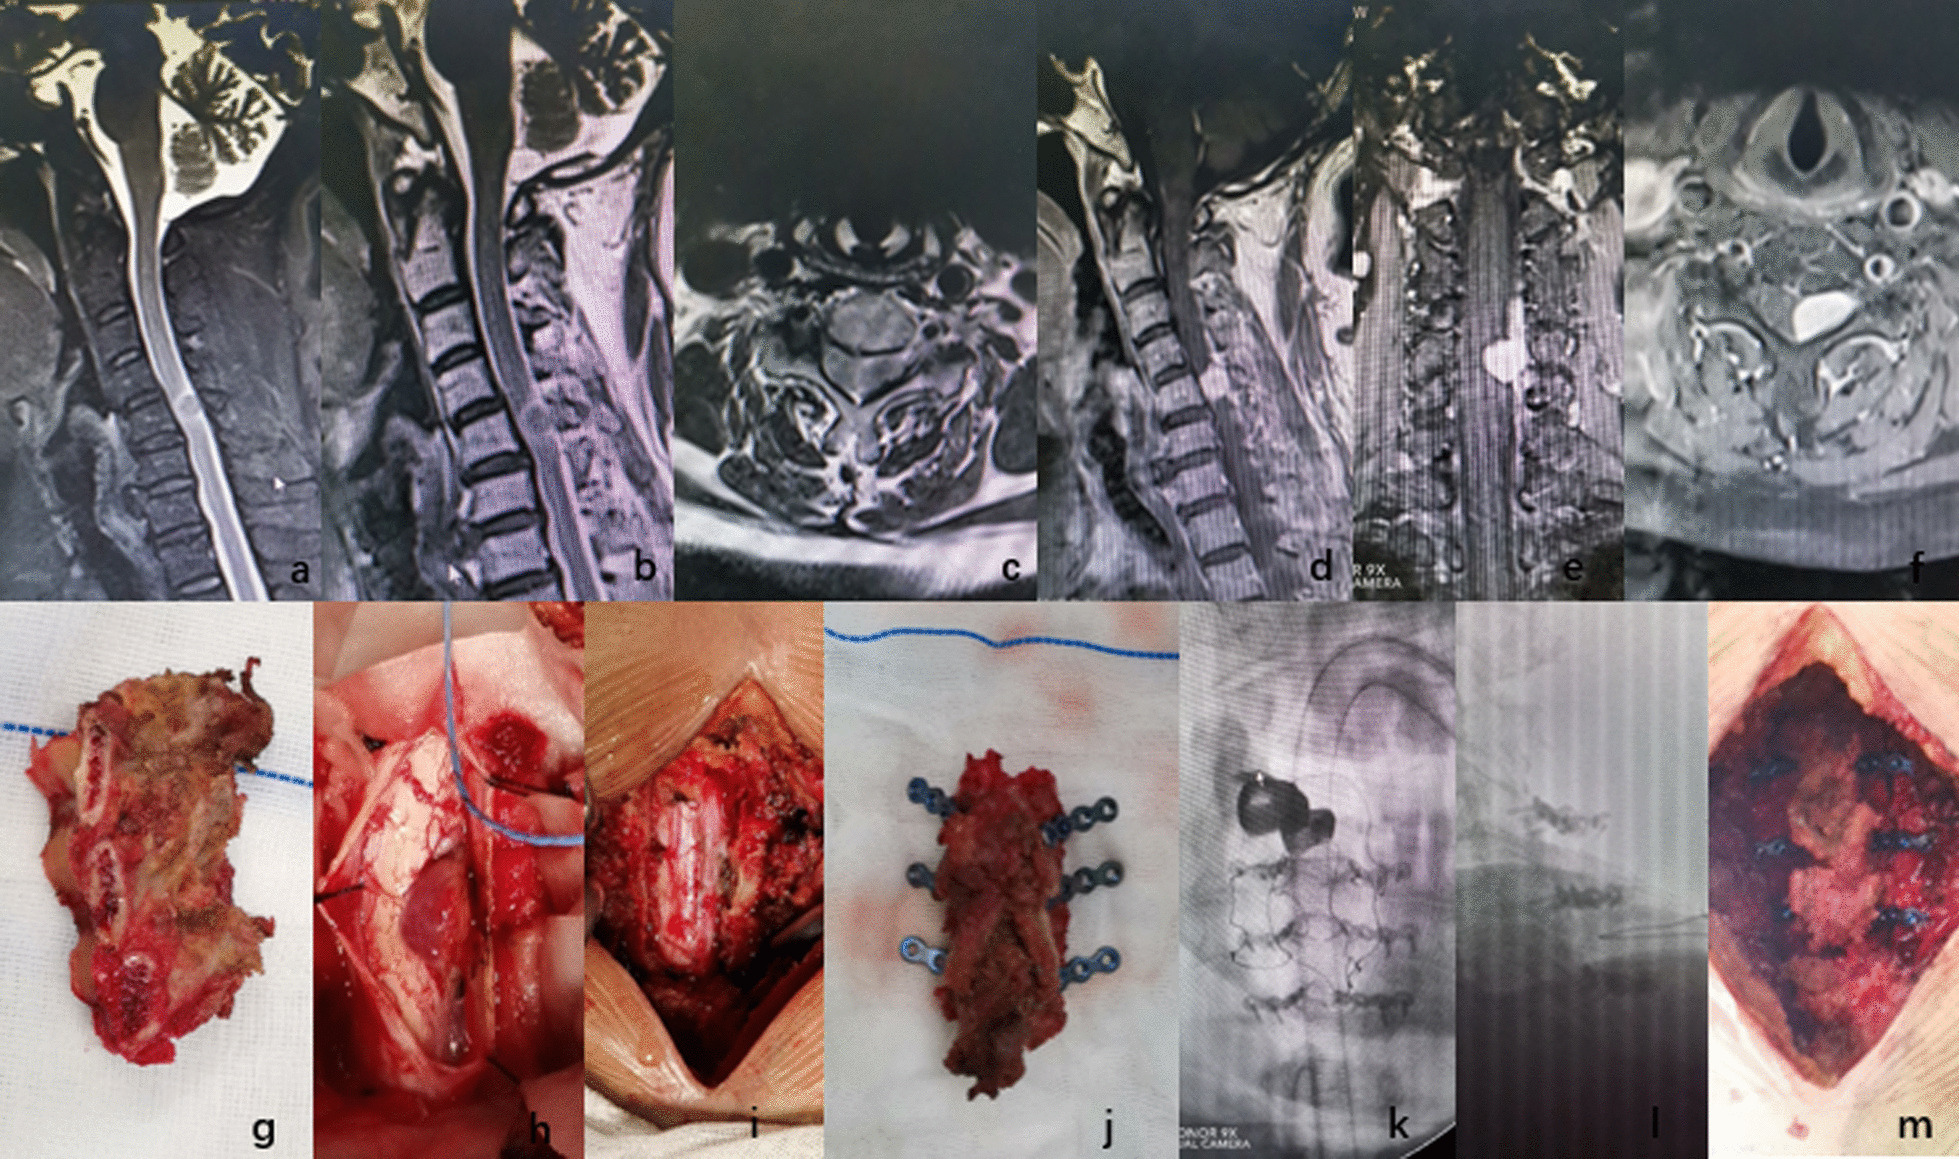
a T2WI image of preoperative MRI examination, b lipid pressure image of preoperative MRI examination, c cross-sectional image of preoperative MRI examination, d sagittal position of preoperative enhanced MRI examination, e coronal position of preoperative enhanced MRI examination, f cross-sectional image of preoperative enhanced MRI examination, g completely removed lamina, h completely exposed tumour, i completely removed tumour, j connected lamina of micro compression locking plate, k sagittal position X-ray after operation, l coronal position X-ray after operation, m completely replanted lamina
